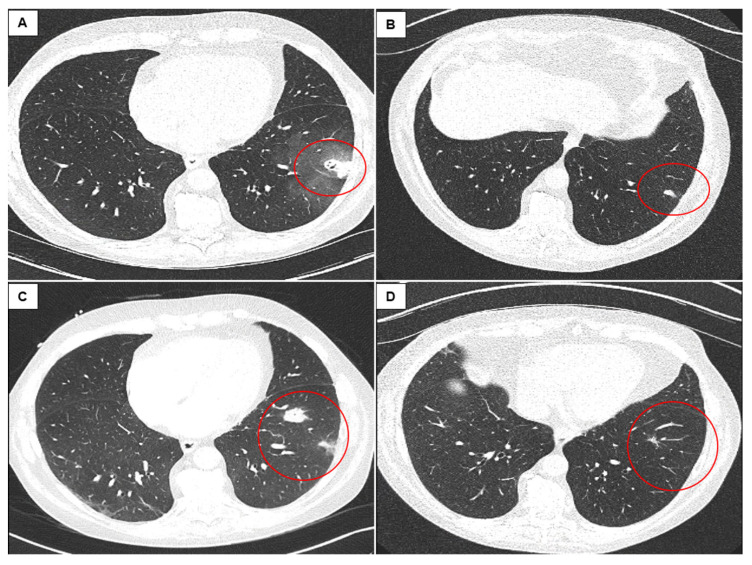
Computed tomography of the chest performed 20 (A) and 89 days (B) after cryobiopsy and forceps biopsy in patient 1 and 16 (C) and 94 days (D) after cryobiopsy and forceps biopsy in patient 2, demonstrating slowly disappearing subsolid and solid consolidations (red circles).
